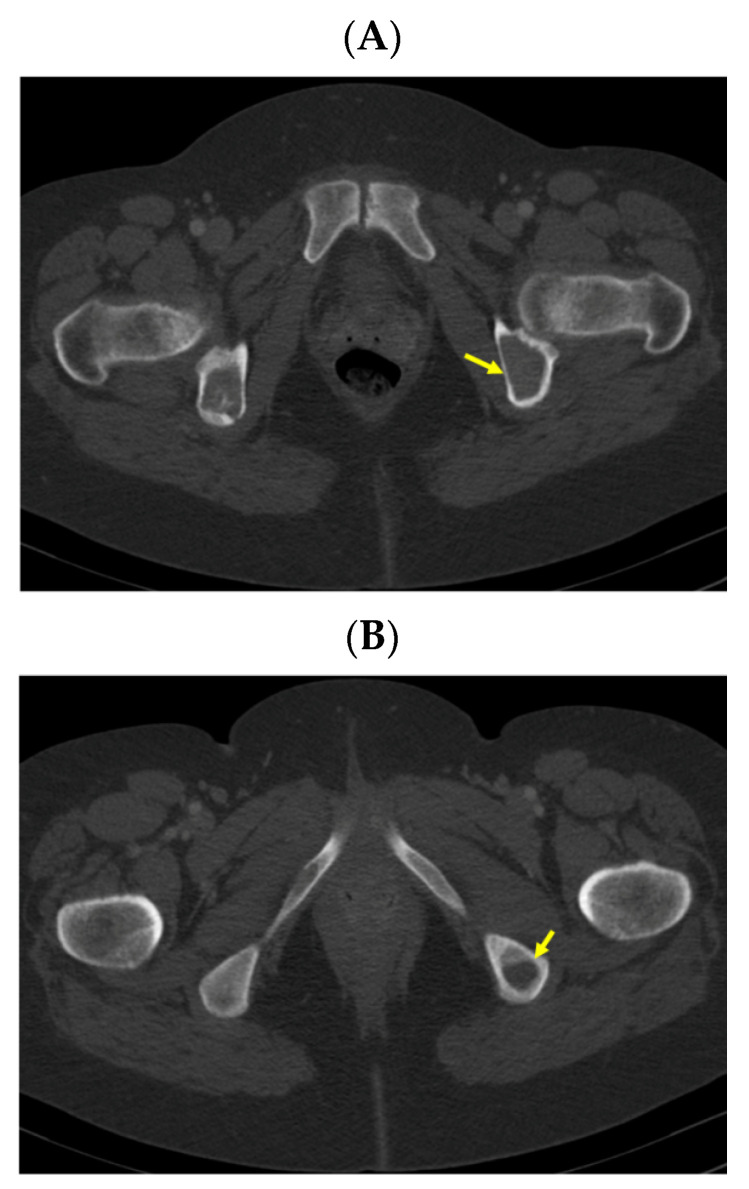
Pelvis CT scans showing other brown tumors: (A) osteolytic lesion of the left coxal bone adjacent to the acetabular fossa of 1.14 by 1.71 cm (axial plane); (B) osteolytic lesion of the left ischium of 1.97 by 3.29 cm (axial plane).
